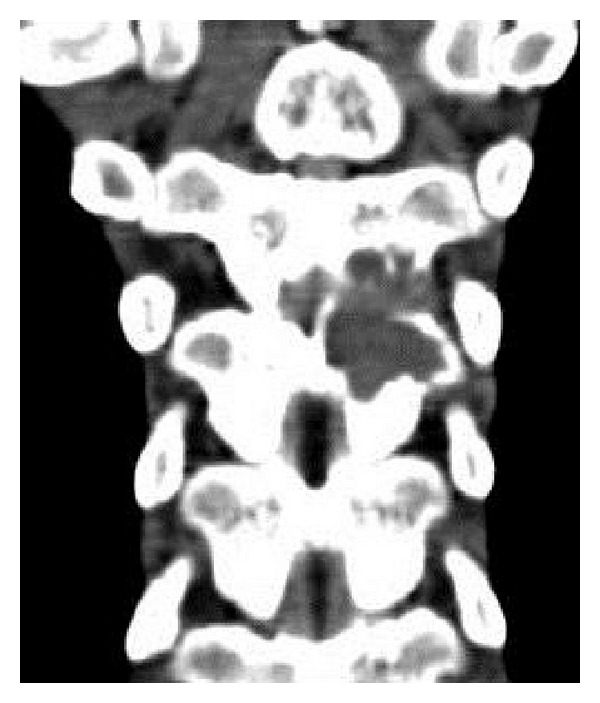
Coronal reformatted CT: eroding areas on the posterior elements of T6 and T7 vertebrae.
